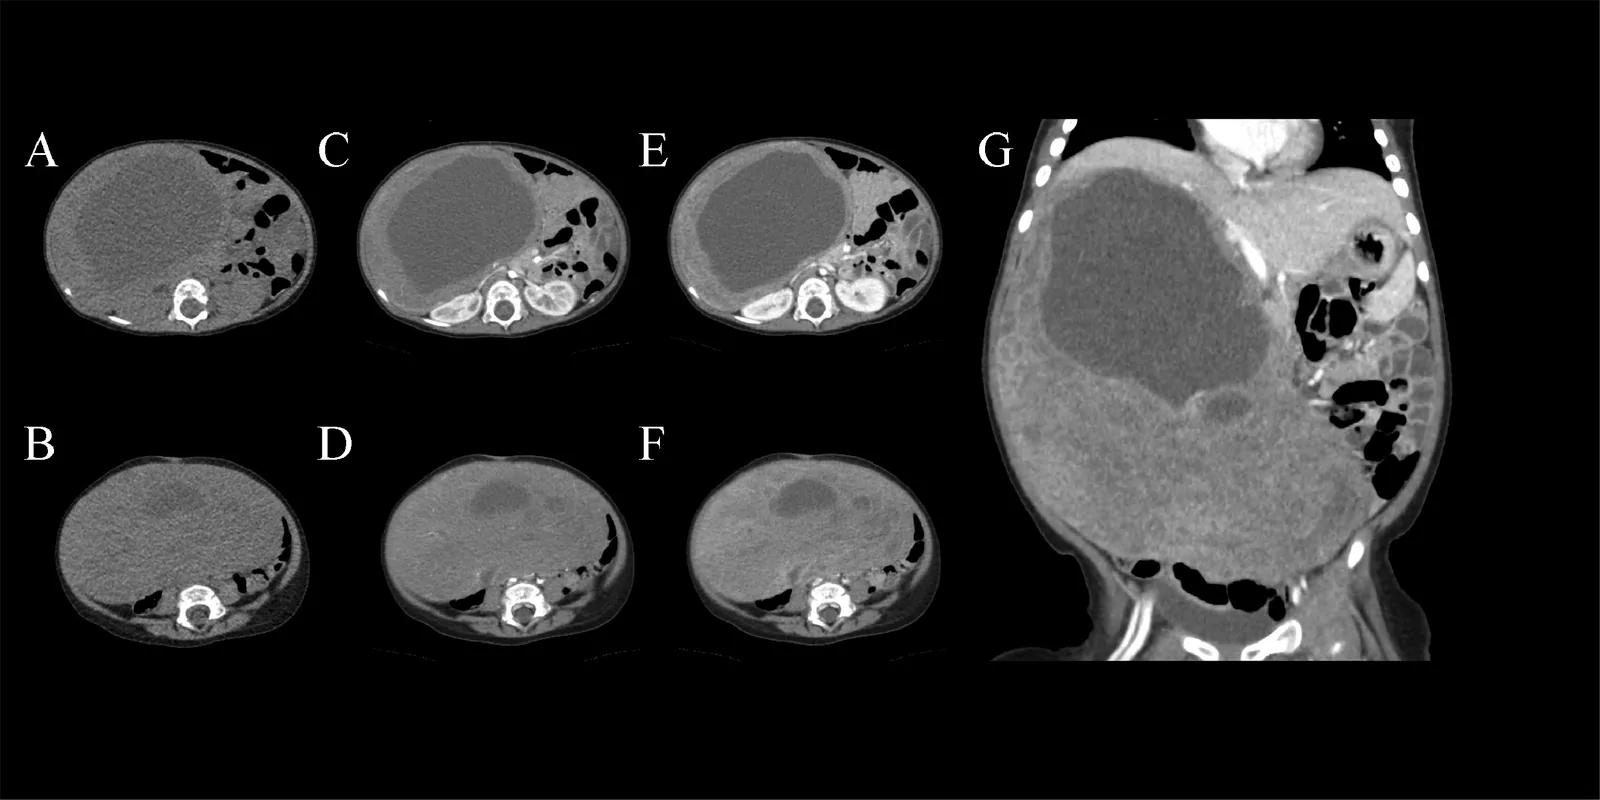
Dynamic contrast-enhanced CT. (A and B): Unenhanced phase, (C and D): Arterial phase, (E and F): Portal phase, (G): Portal phase. Contrast agent was injected at 0.7 mL/s using an injector (for 30 seconds). The arterial phase was captured immediately after injection completion, and the portal phase was captured 20 seconds after contrast agent injection. A large cystic mass extending from the right hepatic lobe into the pelvis was observed. The cystic component measured up to 19 cm in diameter and showed no enhancement. Faint contrast enhancement was noted in the septa and solid portions. No hemorrhage or calcification was observed.
